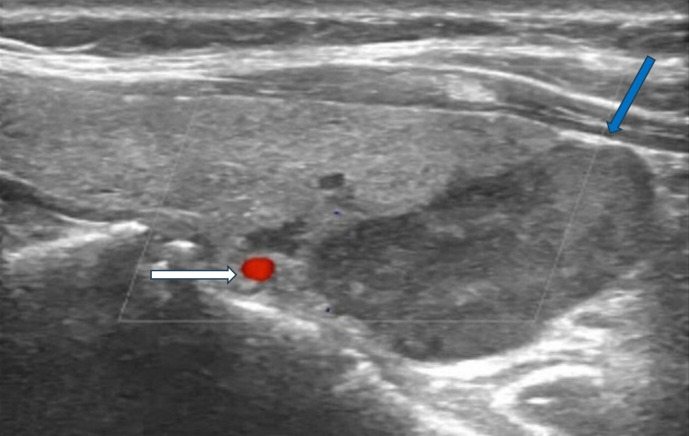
Ultrasonography image of parathyroid carcinoma. Legend: 65-year-old patient with parathyroid carcinoma presented with serum PTH of 1300 pg/mL (12–88 pg/mL) and Ca:13 mg/dL (8.8–10.6 mg/dL). Ultrasonography showed a hypoechoic mass with lobulated borders (32 × 23 × 14 mm) next to the lower pole of the left thyroid lobe (blue arrow). The white arrow shows the inferior thyroid artery.
